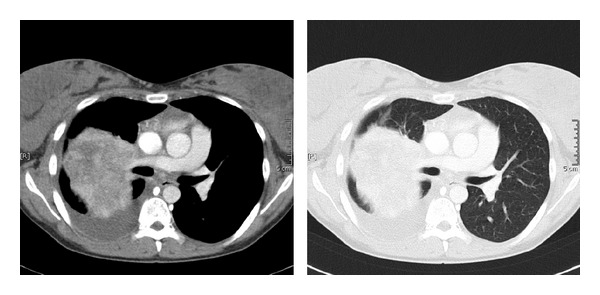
Contrast-enhanced computed tomography revealed pleural infiltration on the right side.
